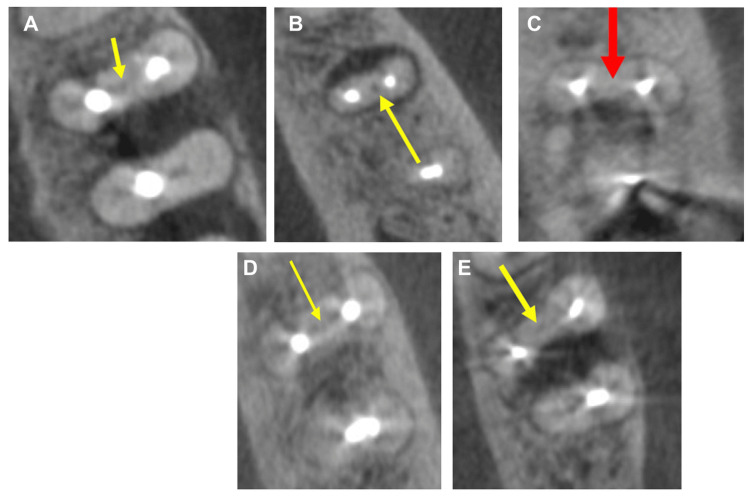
Cone beam images of MMC Panel A. Case 1. This is a right mandibular second molar (tooth 27) in a 25-year-old male, and the distal root had two canals. The left second mandibular molar in this patient was missing, so its status could not be determined. The tooth had undergone endodontic treatment, but the MMC and distal canals had not been treated. The yellow arrow points to missed MMC in the right second mandibular molar. The distal root has two canals. In this case, the left second mandibular molar had been lost. Panels B and C: Case 2. This shows bilateral MMC in a 28-year-old female, where the distal roots had only one canal on both sides. The tooth had undergone endodontic treatment, but the MMC had not been treated. Yellow and red arrows show bilateral missed MMCs, in the left and right second mandibular molars. The distal root has one canal on both sides. Panels D and E: Case 3. This shows bilateral MMC in a 21-year-old female, where both distal roots had two canals. The teeth had undergone endodontic treatment, but the MMC had not been treated. There was no notable association between the existence of MMC in the mesial root and a second distal canal in the same molar tooth. Yellow arrows show missed MMCs in the left and right second mandibular molars. The distal root has two canals on both sides. MMC, middle mesial canal
